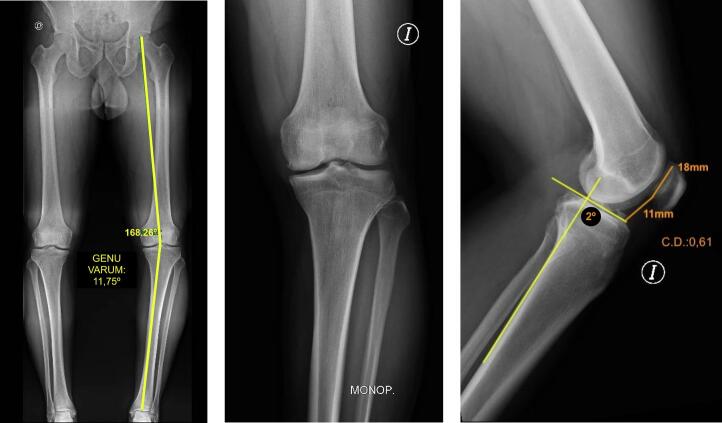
Preoperative measurements: A) Long-leg alignment radiograph showing genu varum. B) Anteroposterior monopodal weight-bearing radiograph. C) Measurement of posterior tibial slope and Caton-Deschamps index.
